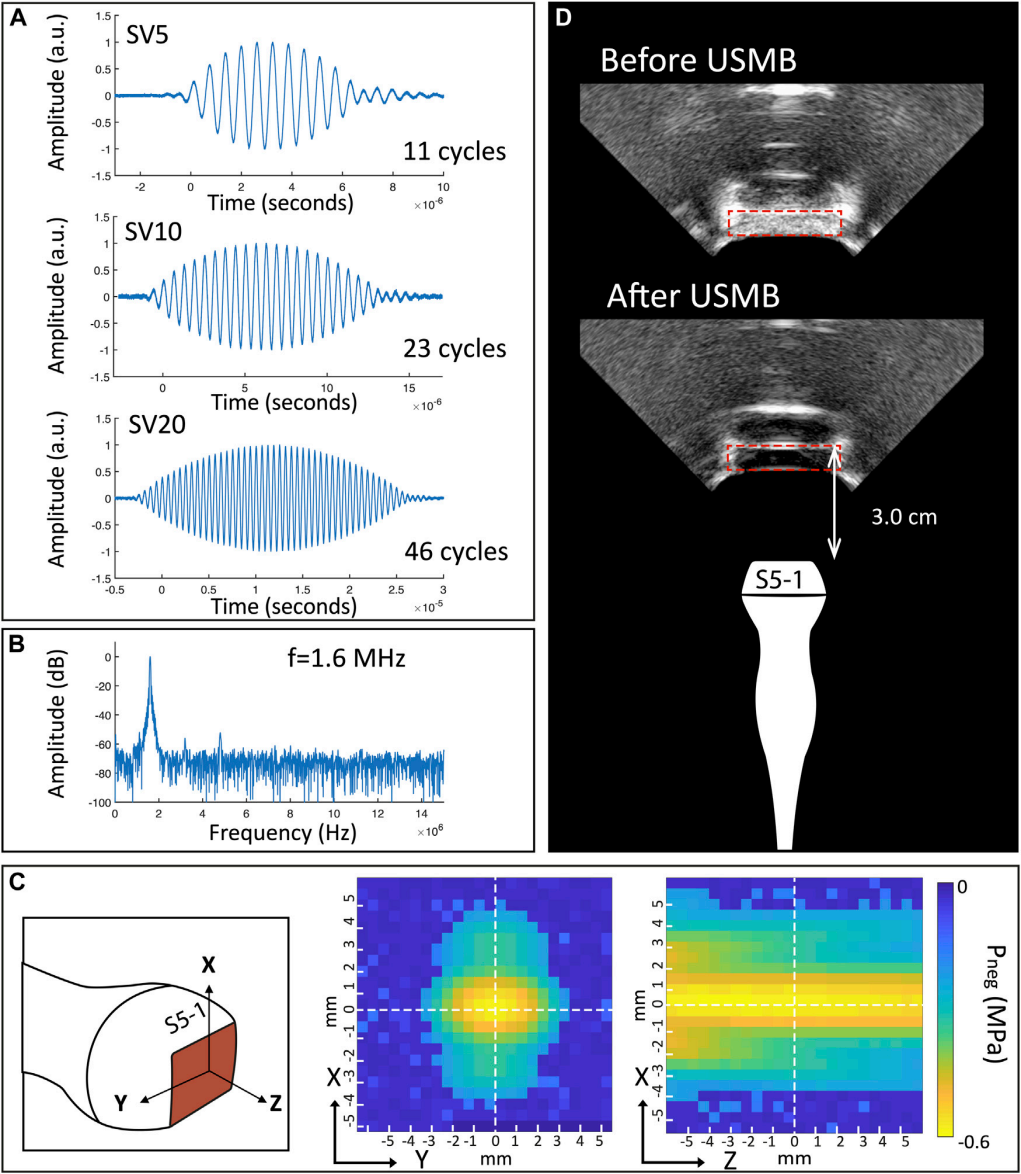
Characteristics of S5-1 probe (A) Shape of emitted ultrasound pulses in PW mode for different pulse length. (B) Frequency spectrum of SV 20 mm pulse. (C) Pressure field maps in PW mode. (D) B-mode images of TwentiCell (red rectangle) containing microbubbles, before and after USMB therapy (15 s at MI 0.6, SV 20 mm). SV: sample volume; f: frequency; a.u.: arbitrary units; P_neg_: Peak negative pressure; USMB: ultrasound and microbubbles.
